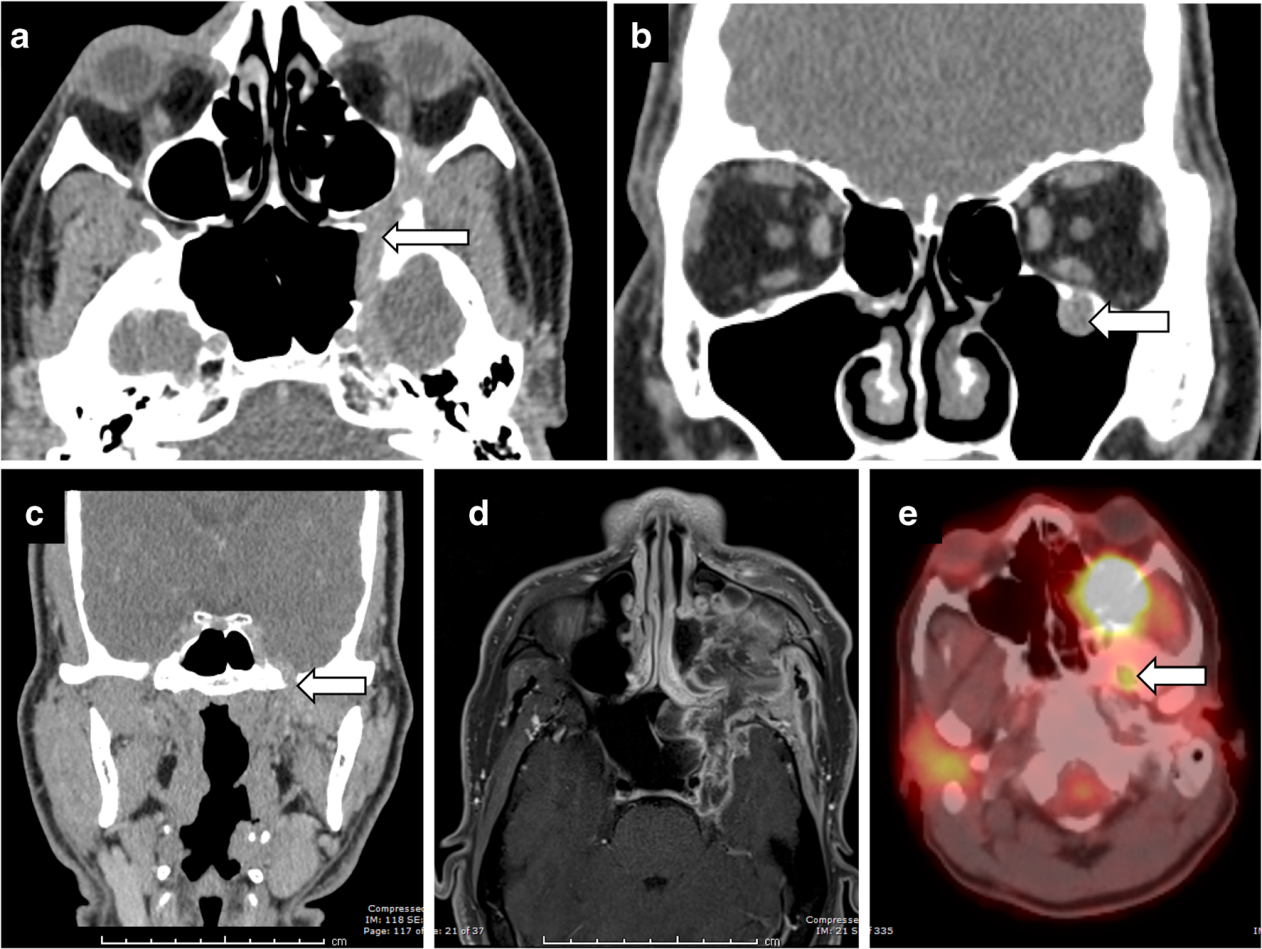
Perineural spread of tumour. A 45-year-old man with left facial pain and numbness. CT showing enhancing soft tissue along the course of left CN V, expanding into the foramen rotundum (a), infraorbital foramen (b) and foramen ovale (c). Posteriorly, the enhancing tumour extends to the left Meckel’s cave. Rapid expansion on follow-up MRI (d) with intense uptake on positron emission tomography (PET) (e) in left CN V including foramen ovale (*arrow*)
